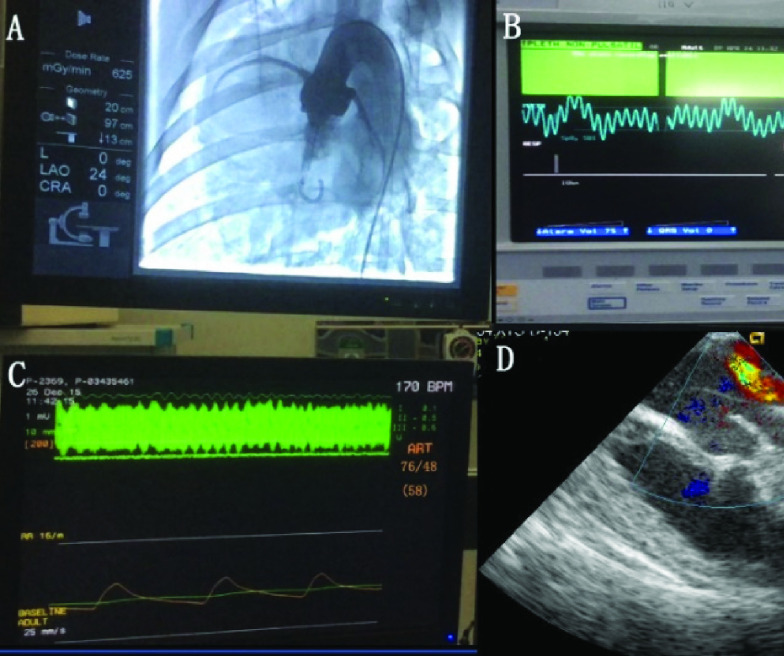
After starting the left ventricular assist device, blood was pumped from the left ventricle to the ascending aorta, with good coronary artery filling (a). Blood oxygen saturation at the peripheral body surface was 100% (b). The pressure in the carotid artery was 76/48 mmHg (c). No serious aortic valve reflux was observed on color Doppler imaging (d).
